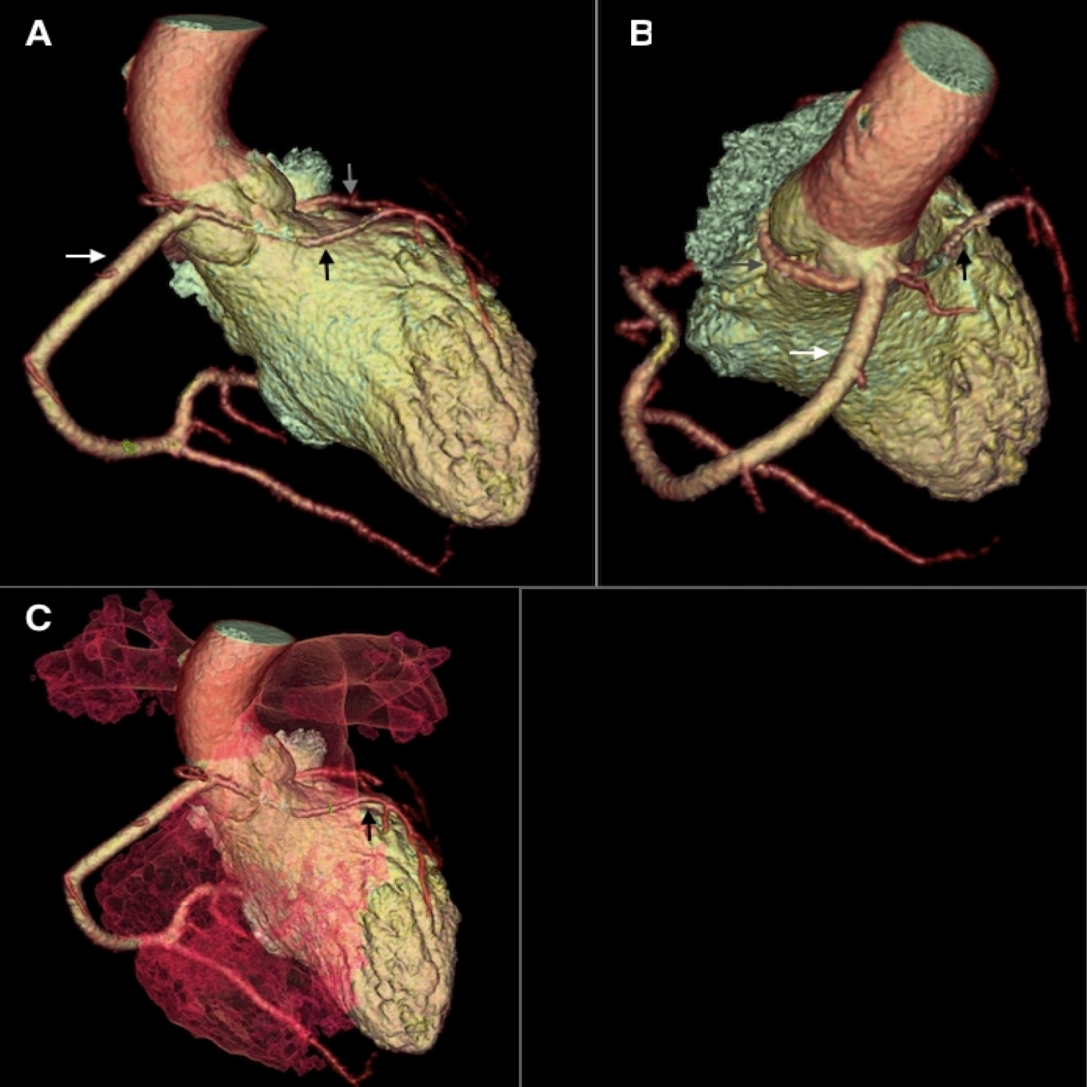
(A) A volume rendered 3D computed tomography (CT) angiogram (anterior view) showing a large dominant right coronary artery (white arrow) arising from right coronary sinus and supplying most area of the myocardium of heart. The right coronary artery measures about 5 mm proximally. Left anterior descending artery (black arrow) appears to be originating from right coronary sinus and is a small vessel (1.7 mm at its greatest diameter). Small left circumflex artery (gray arrow) is also seen in the image posterior to the aorta. (B) A volume rendered 3D CT angiogram (posterior view) demonstrating origin of left anterior descending artery (black arrow), left circumflex artery (gray arrow) and right coronary artery (white arrow) from right coronary sinus. Small anomalous left circumflex artery has a benign course running posterior to aorta and then coming into posterior atrioventricular groove. (C) A volume rendered 3D CT angiogram (anterior view including right ventricle and pulmonary arteries) shows a malignant course of left anterior descending artery (black arrow) running between ascending aorta and pulmonary trunk.
